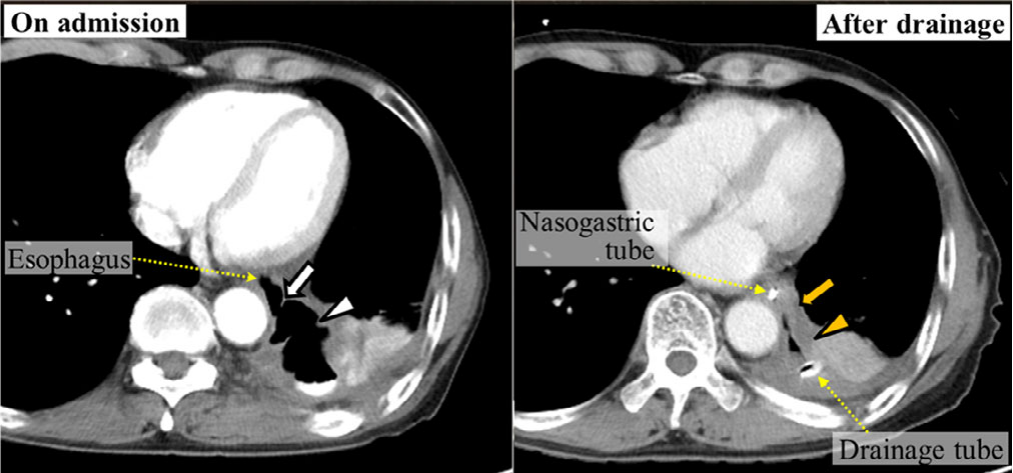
Contrast‐enhanced computed tomography on admission and after preoperative abscess drainage. On admission, the fistula in the left wall of the thoracic lower esophagus (white arrow) was continuous with an abscess in the left thoracic cavity (white arrowheads). After preoperative abscess drainage, the fistula (orange arrow) and abscess cavity (orange arrowheads) had both decreased in size
